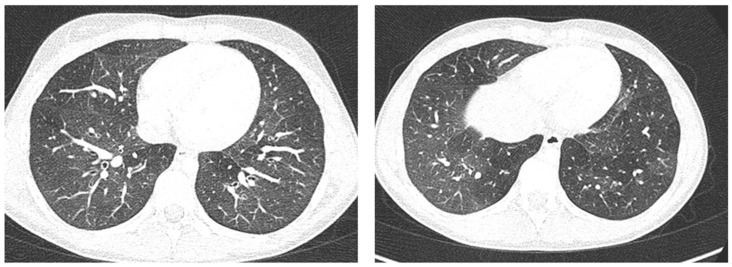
A representative coronal section from thoracic computed tomography (CT) scan reveals: thickening of the bronchial walls bilaterally, with filling of the bronchial lumen predominantly in the apical segment of the right upper lobe; picture of diffuse inhomogeneity of parenchymal density with diffuse and bilateral hypodense areas in relation to air trapping correlated to a picture of recurrent airway inflammation.
